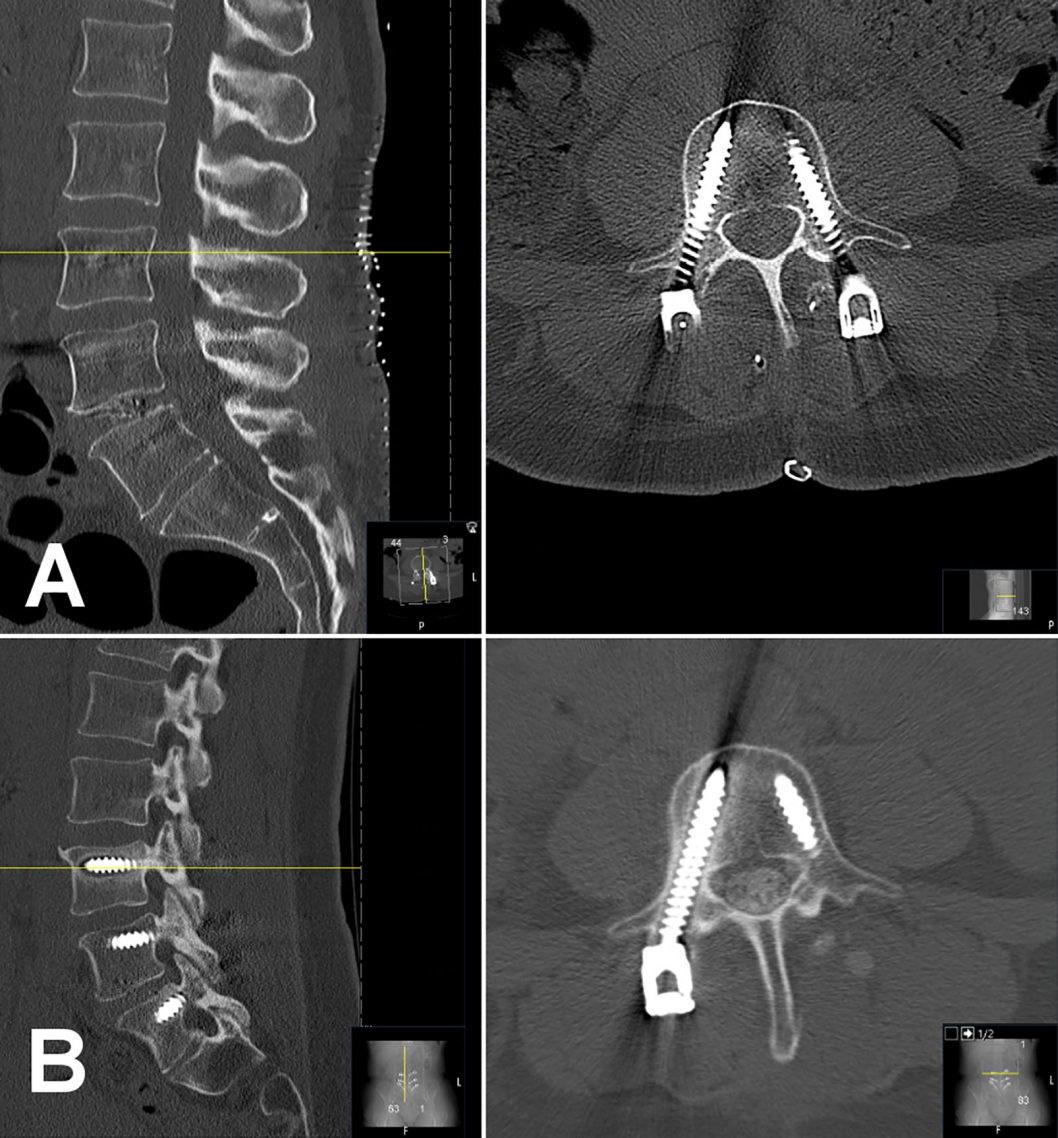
Case of a 48-year-old woman who underwent spinal surgery with semi-rigid instrumentation of levels L4 to S1 for lumbar instability. Of note, this patient has a partially lumbarized S1 vertebra that shows bony fusion at the lateral mass to the os sacrum. Opportunistic BMD evaluation yielded low bone mass (BMD = 95.8 mg/cm³). An immediate postoperative CT scans shows all six pedicle screws at L4, L5, and S1 and an intervertebral cage at L5/S1 in place (A). In 3-months survey the patient reported severe disability according to Oswestry Disability Index (score 42). A control CT scan 178 days after index surgery showed loosening of right L4 screw and both S1 screws (screws not fully depicted; B). BMD, bone mineral density.
